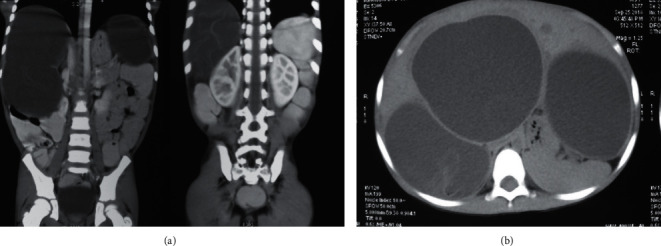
Abdominal CT scan showing multiple cysts with floating membranes in hepatic segments 2, 3, 6, and 7 with features favoring hydatid etiology.
